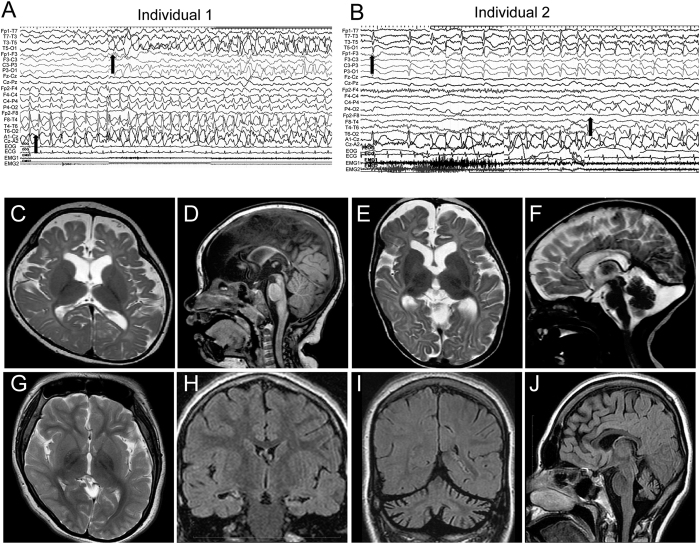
Clinical features of individuals with biallelic *SLC12A5* mutations. (A) Ictal EEG of individual 1. The initial spikes over the right frontal area (lower arrow) were accompanied by eye deviation to the left, then the left temporal spikes emerged (upper arrow) with subsidence of the right frontal spikes, which were accompanied by eye deviation to the right. (B) Ictal EEG of individual 2. The initial spikes over the left temporal area (upper arrow) were accompanied by a tonic seizure of the right upper extremity, then the right parietal spikes emerged (lower arrow) with subsidence of the left temporal spikes, which was accompanied by a tonic seizure of the left upper extremity. (C–J) Brain MRI of individual 1 at 13 months of age (C,D), individual 2 at 5 months (E,F), and individual 4 at 20 years of age (G–J). T2-weighted images (C,E–G) and T1-weighted images (D,J) and fluid-attenuated inversion recovery images (FLAIR) (H and I) are shown. Thin corpus callosum, frontal and temporal lobe atrophy, and delayed myelination were commonly observed in individuals 1 and 2 (C–F). Arachnoid cyst in the left posterior fossa was observed in individual 2 (F). Delayed myelination in the subcortical white matter of the temporal lobe was observed in individual 4 (G). Inferior horns of the lateral ventricle were mildly dilated and bilateral hippocampi were hypoplastic with slightly high signal intensity on FLAIR coronal view (H), indicating hippocampal sclerosis. Atrophic change of the cerebellar hemisphere (I) and vermis (J) was evident.
